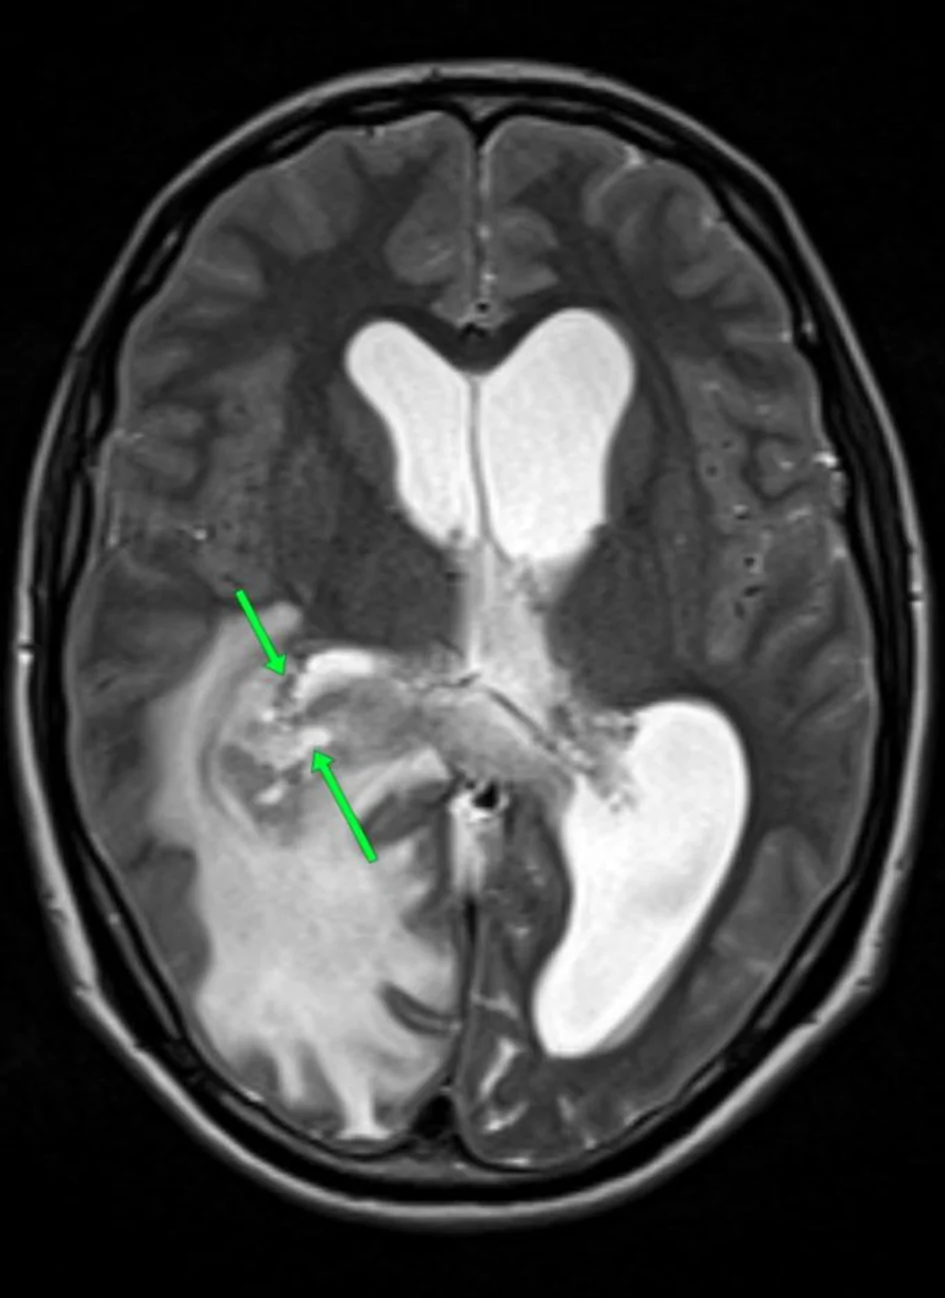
Axial T2-weighted MRI demonstrating a heterogeneous lesion with multiple septations and mixed cystic and solid components, without diffusion restriction on DWI. DWI: diffusion-weighted imaging; MRI: magnetic resonance imaging.
